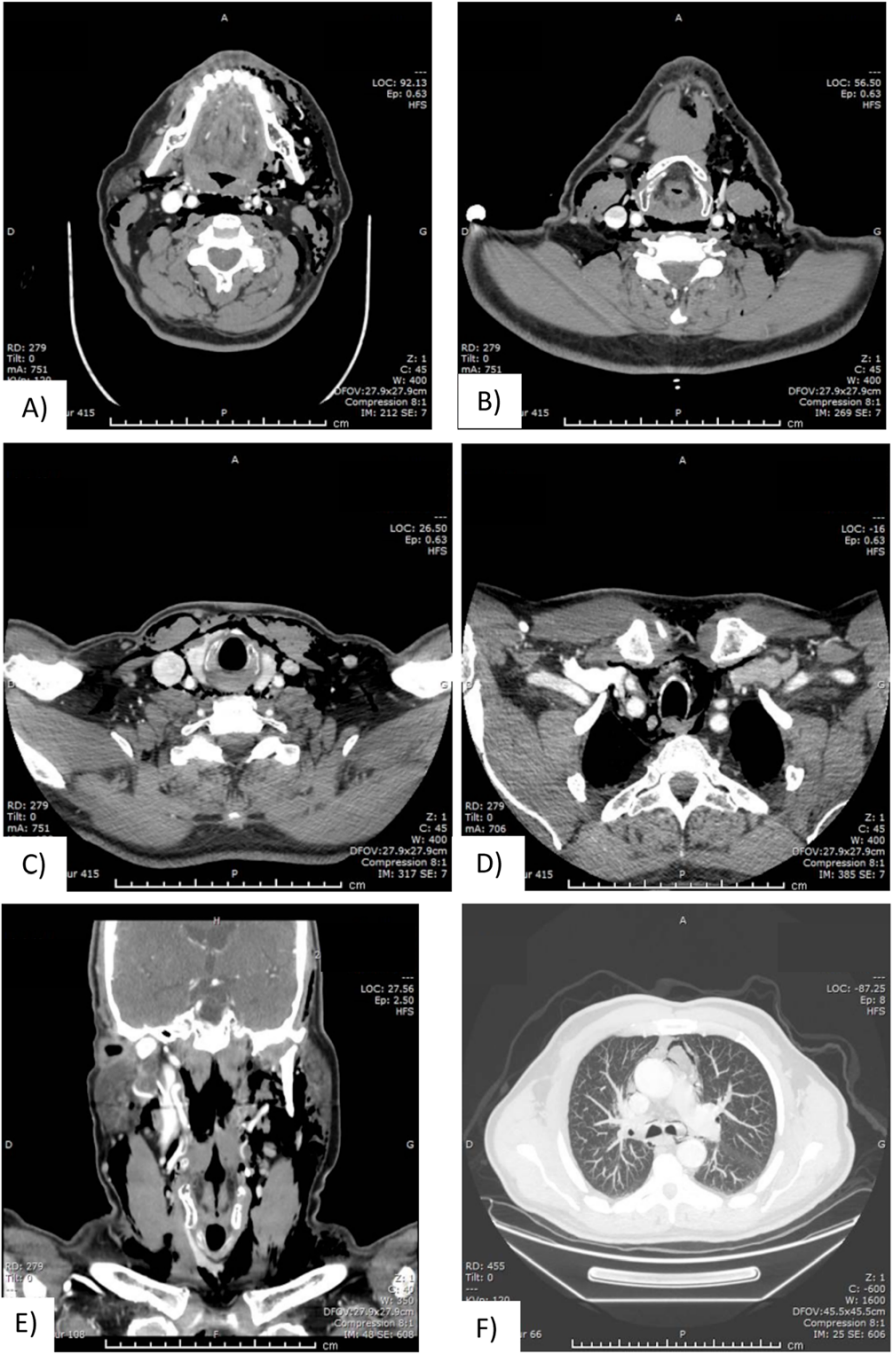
Cervicothoracic CT on arrival. a through f) Extensive emphysema, involving almost every deep neck and mediastinal spaces
